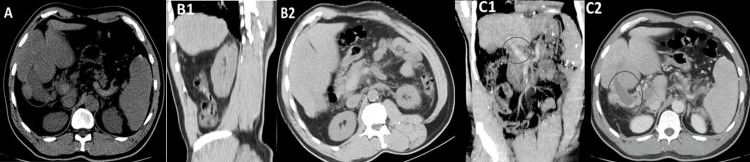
Abdominopelvic CT with intravenous contrast (A) Axial plane, without contrast. Portal vein with a hyperdense thrombus (circle), indicating that it is recent. (B) Atrophy of the right lobe of the liver (arrows) with no other signs of chronic liver disease - (B1) sagittal plane; (B2) axial plane. (C) Portal vein thrombosis (circle). Perivascular venous collaterals and at the level of the hepatic hilum (arrows); (C1) coronal plane; (C2) axial plane.
